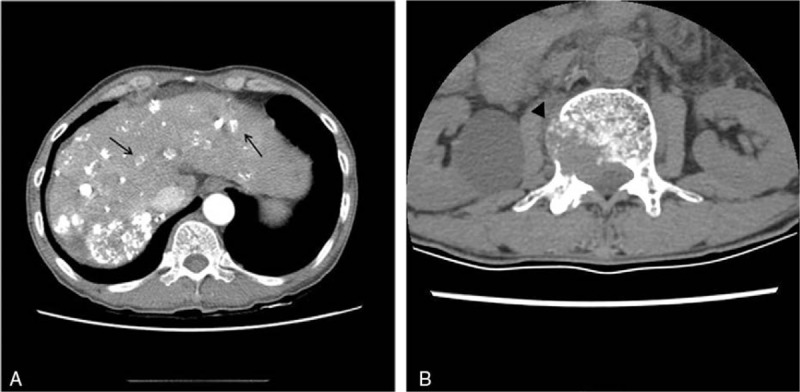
Computed tomography images of the abdomen and lumbar spine show multiple lipiodolized arterial enhancing hepatocellular carcinomas (arrow) in the cirrhotic liver after transcatheter arterial chemoembolization (A), and bony metastasis at the body of the second lumbar spine (arrowhead) (B).
